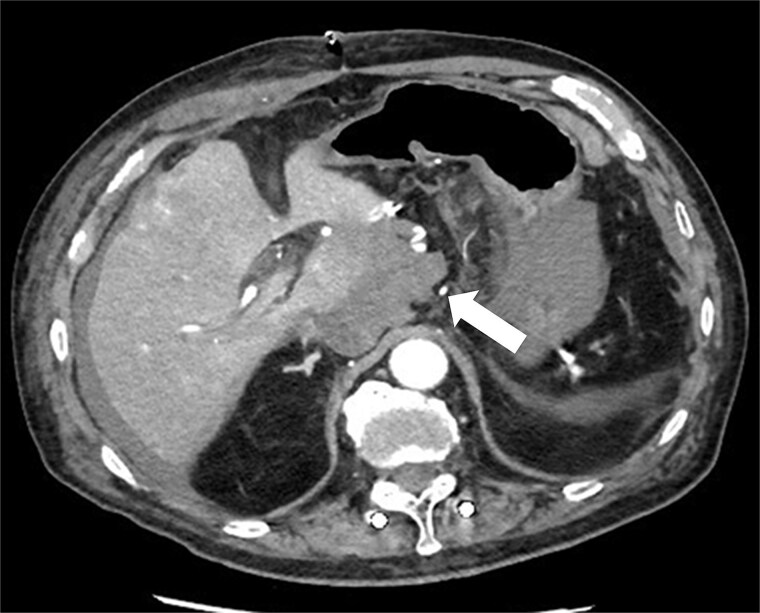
POD 1 contrast-enhanced CT demonstrating a hematoma with active contrast extravasation near the Spiegel lobe (arrow).
